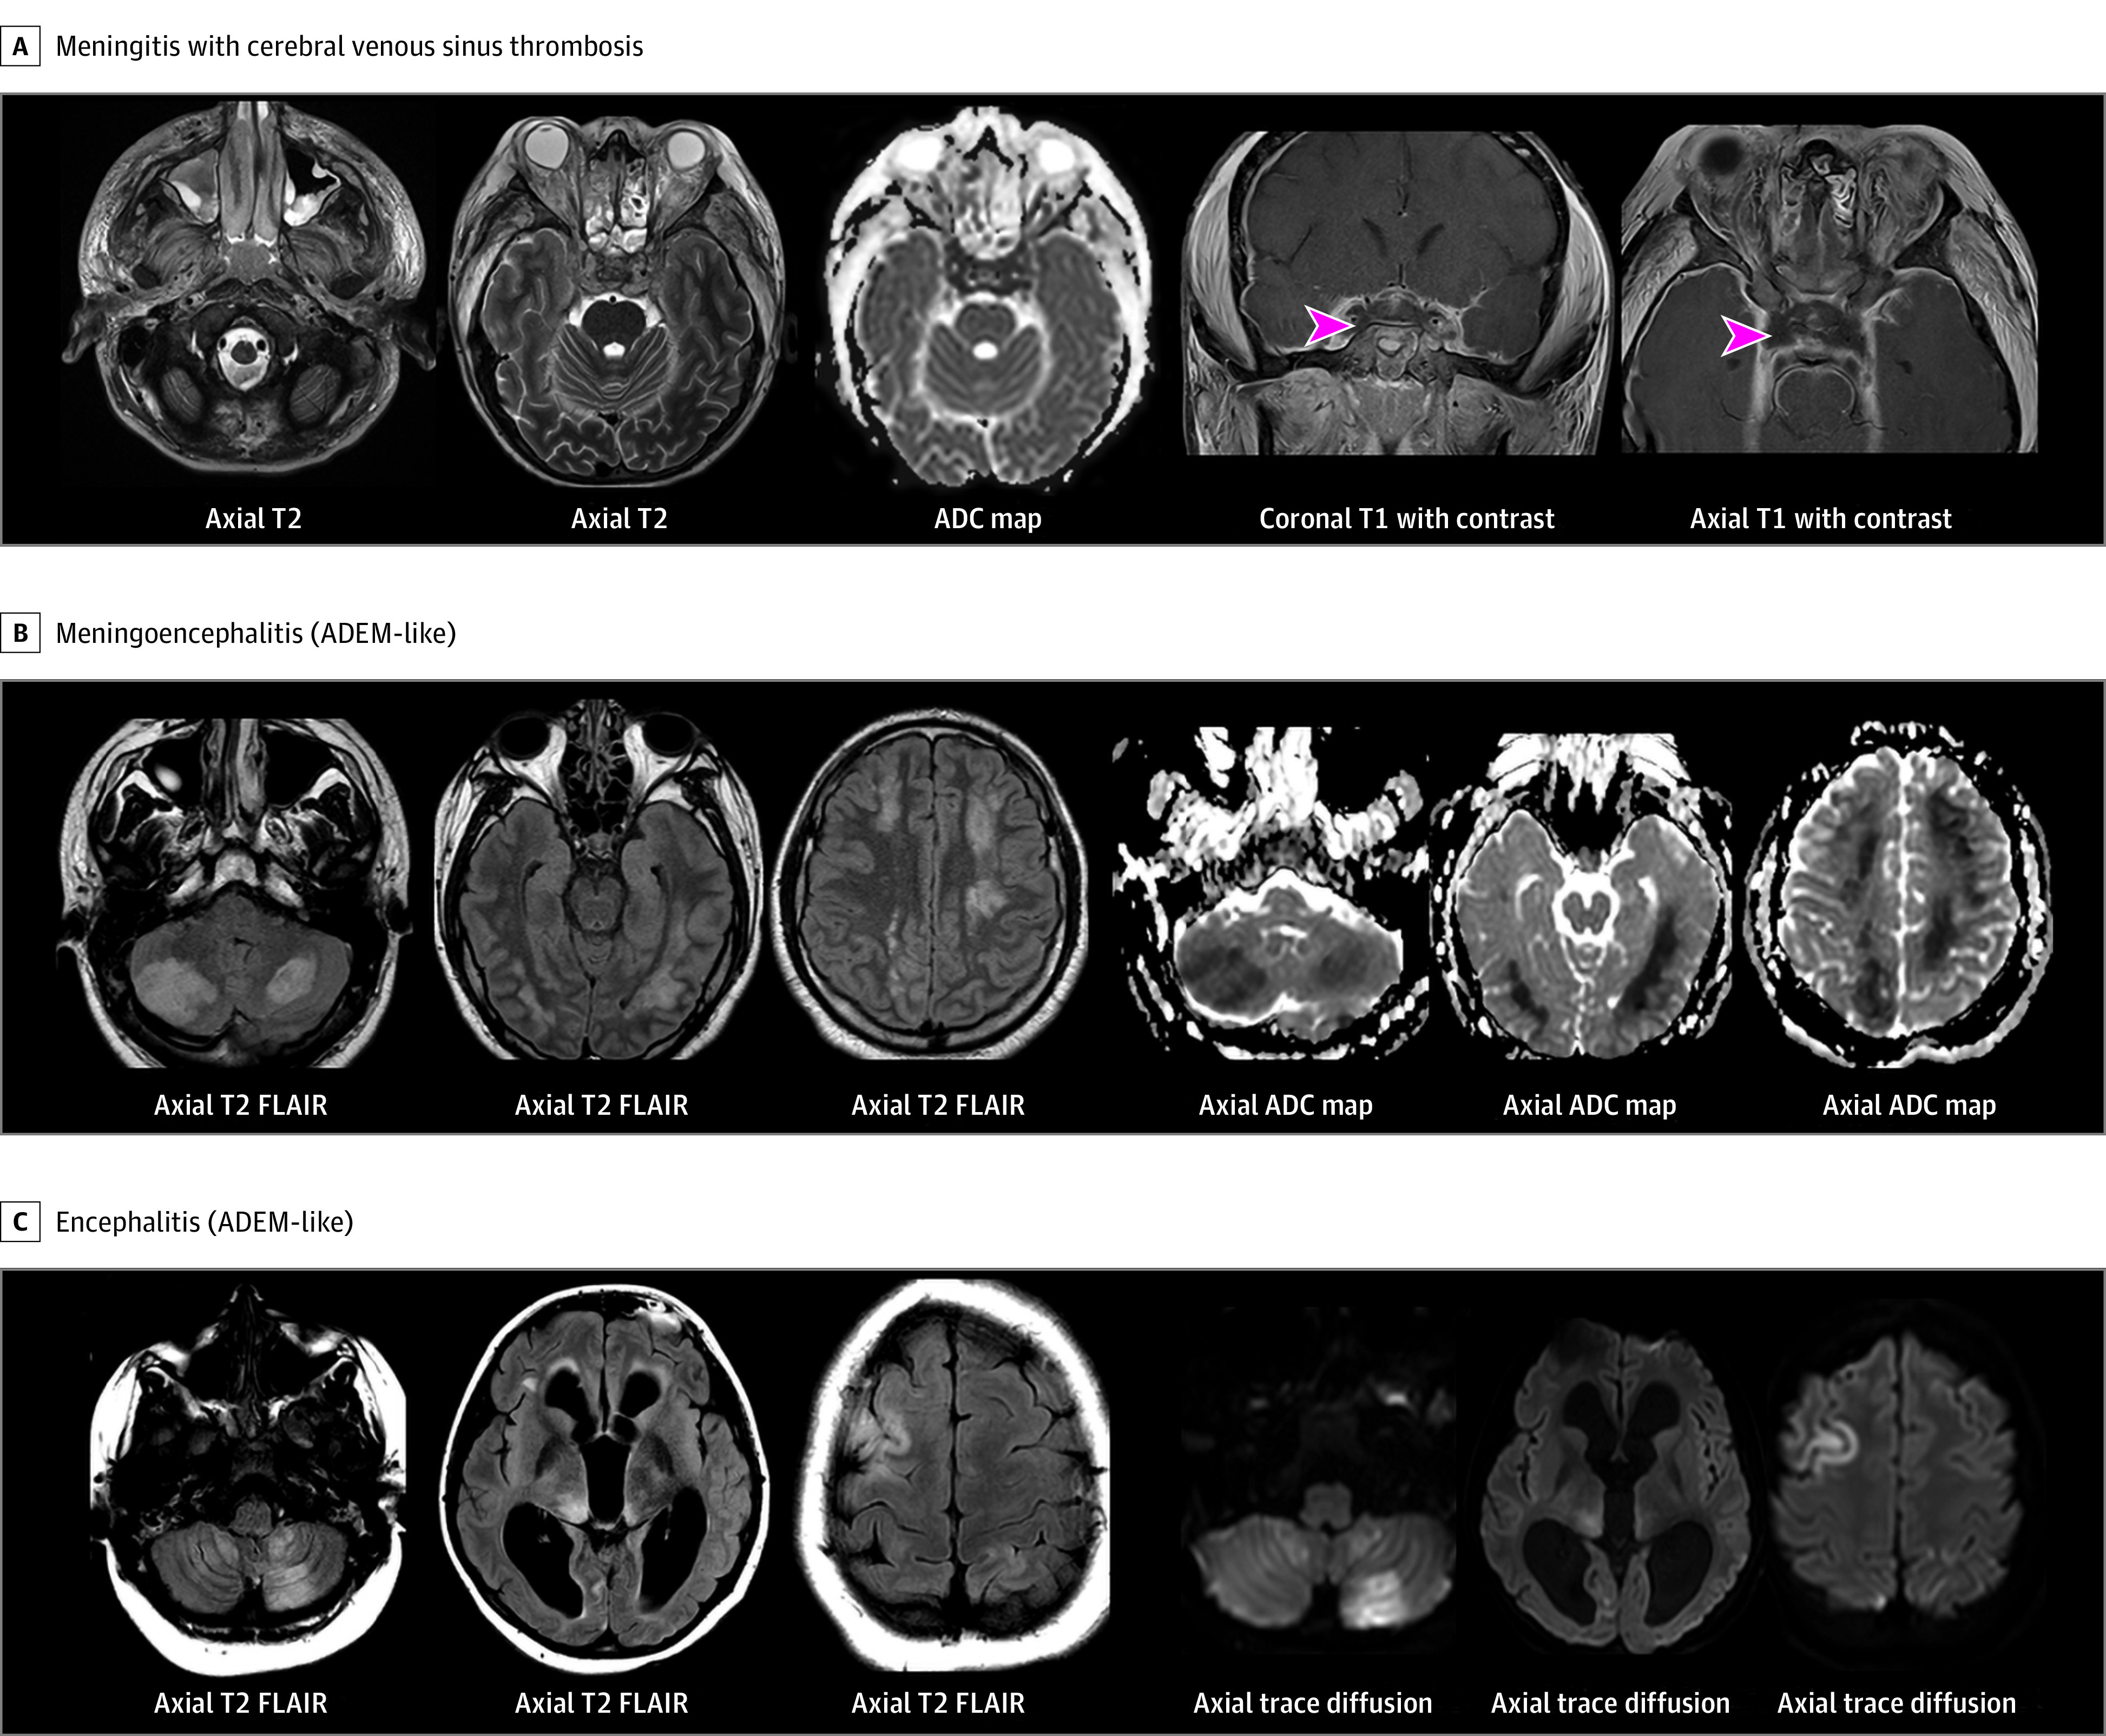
Representative Central Nervous System Images From Patients With Life-threatening COVID-19–Related Neurologic Involvement A, Teenager presented with acute respiratory failure, fever, acute onset of altered awareness, confusion, agitation, and difficulty walking. Axial T2 images show myositis of the facial muscles with stranding of subcutaneous fat, sinusitis, and cavernous sinus thrombosis with T2 hypointense signal and reduced diffusivity (apparent diffusion coefficient [ADC] map). Coronal and axial T1 images with contrast demonstrate dural enhancement, filling defects in superior ophthalmic veins and cavernous sinuses consistent with thrombosis (arrowheads). The patient’s brain injury evolved to diagnosis of death by neurologic criteria. B, Teenager with history of obesity, hypertension, and diabetes presented with 3 weeks of fever, confusion, headache, seizures, orofacial dyskinesias, agitation, slurred speech, difficulty walking, chorea, and left-sided weakness. Axial T2 fluid-attenuated inversion recovery (FLAIR) images demonstrate T2 hyperintense signal in bilateral cerebellar, bilateral temporal, and bilateral centrum semiovale white matter with reduced diffusivity. There was no enhancement, and susceptibility was present in lesions consistent with punctate blood products (not shown). The patient later died by brain death. C, Teenager presented with 3 weeks of fever, headaches, lethargy, confusion, seizure, vomiting, blurry vision, and nystagmus. Axial T2 FLAIR images demonstrate moderate enlargement of lateral and third ventricles, T2 prolongation in bilateral cerebellar hemispheres, bilateral thalami, and bifrontal white matter and cortex with reduced diffusivity on trace diffusion images and no enhancement (not shown). ADEM indicates acute disseminated encephalomyelitis.
